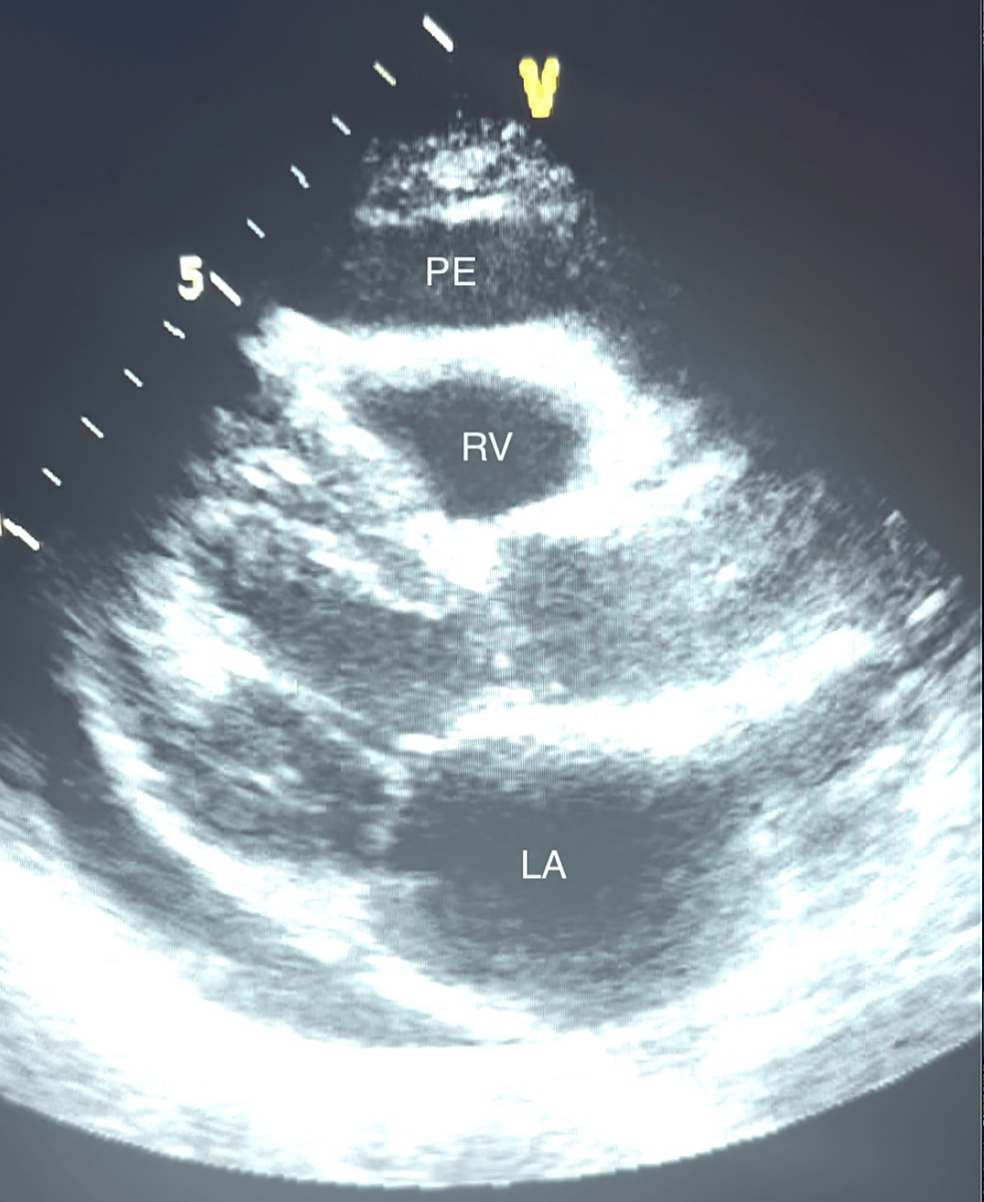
Parasternal long-axis view on TTE revealing right ventricular collapse as a result of pericardial effusion LA: Left atrium; PE: pericardial effusion; RV: right ventricle; TTE: transthoracic echocardiogram
